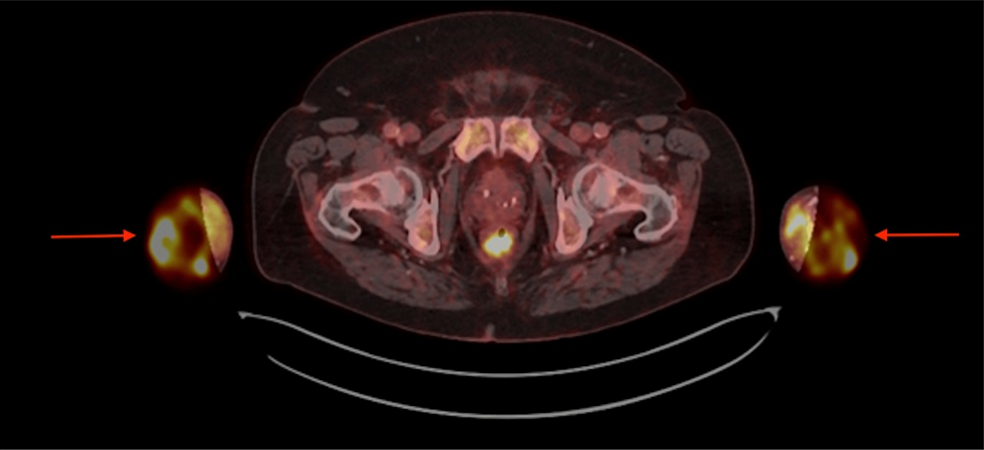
F-18-FDG PET/CT scanning with contrast uptake from both wrists (arrows) F-18-FDG: Flour-18-Fluorodeoxyglucose; PET/CT: positron emission tomography/ computed tomography
